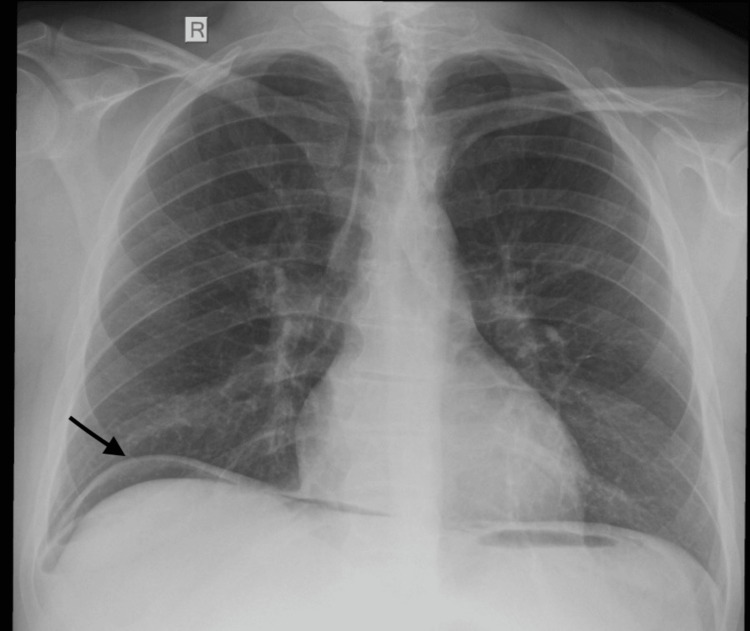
Free gas in x-ray (arrow), indicating perforation
